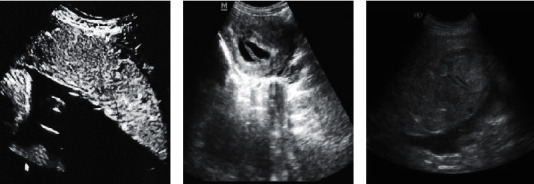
Ultrasound image characteristics of dangerous placenta previa.
